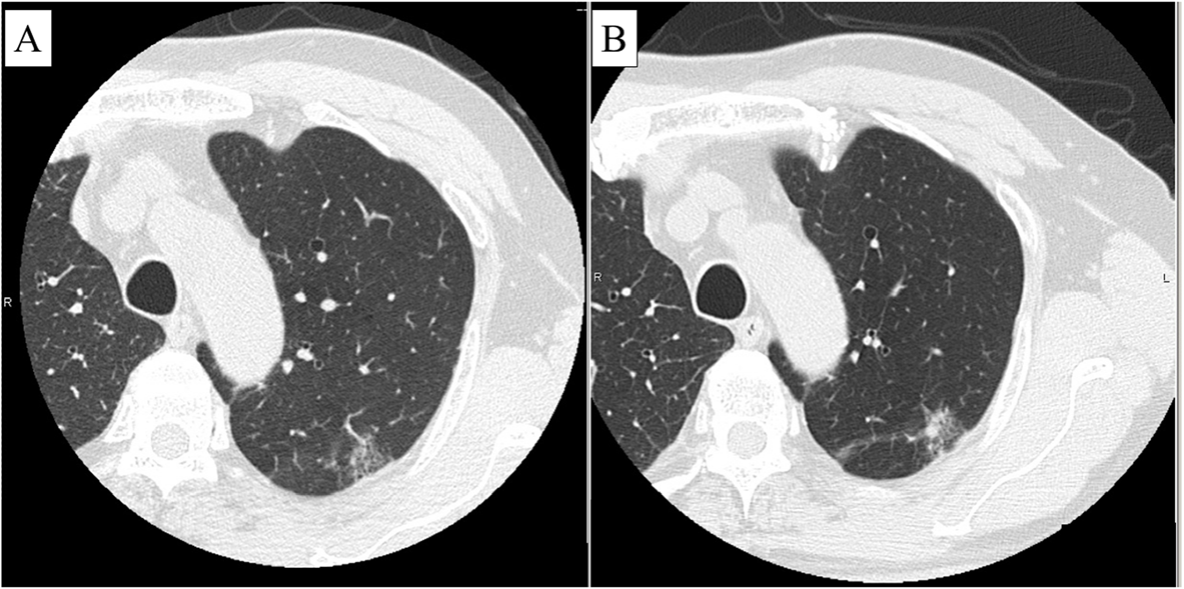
Preoperative chest computed tomography images. a Initially, chest computed tomography showed a pure ground-glass nodule with a possibility of benignity. b Six months later, chest computed tomography revealed a part-solid ground-glass nodule containing a solid component that was highly suspicious for malignancy
